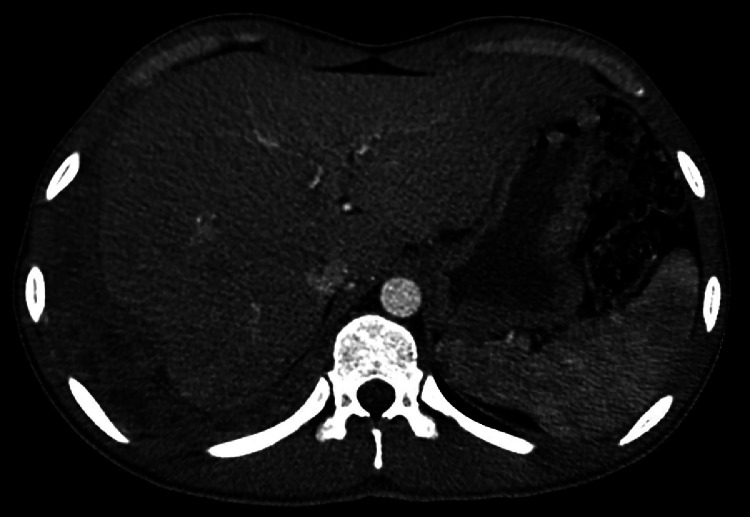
CT scan of the abdomen. Imaging revealing right pleural thickening with loculated low-attenuation areas and rim enhancement, extending to the diaphragm and the liver capsule, suggestive of multiloculated abscess/empyema from tuberculosis.
